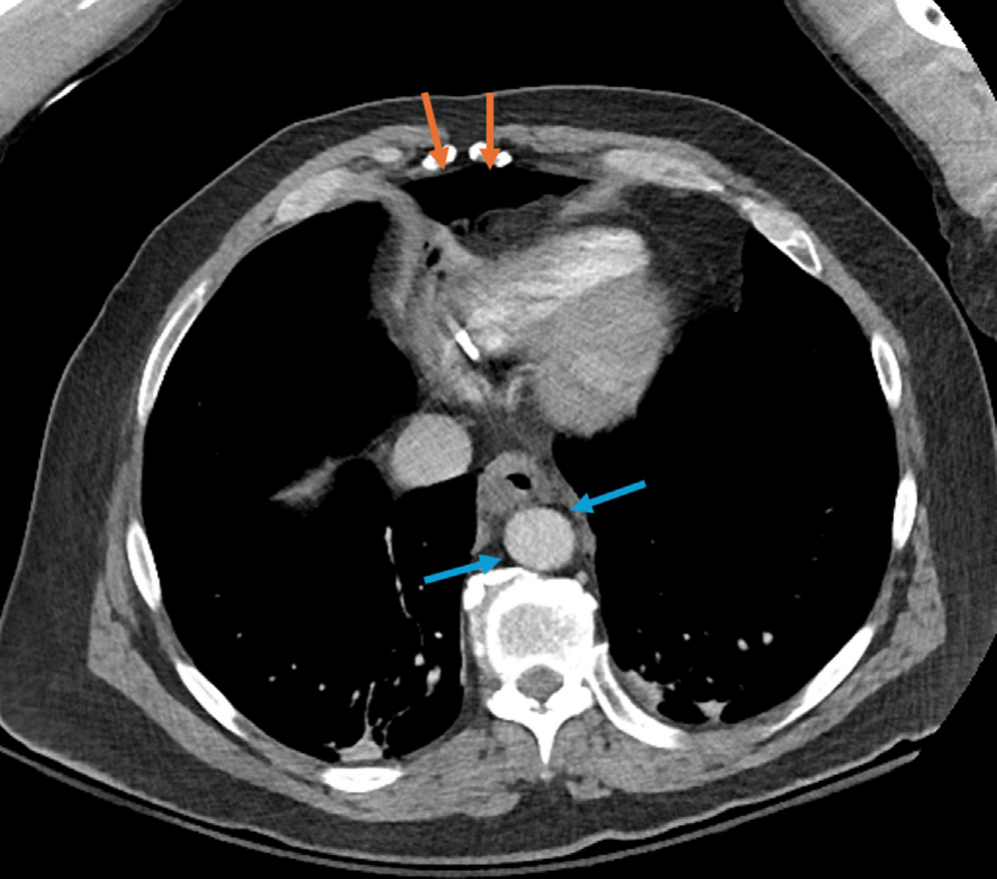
Computed tomography of the chest with intravenous contrast demonstrating pneumomediastinum (*orange arrows*) and retroperitoneal air (*blue arrows*).
